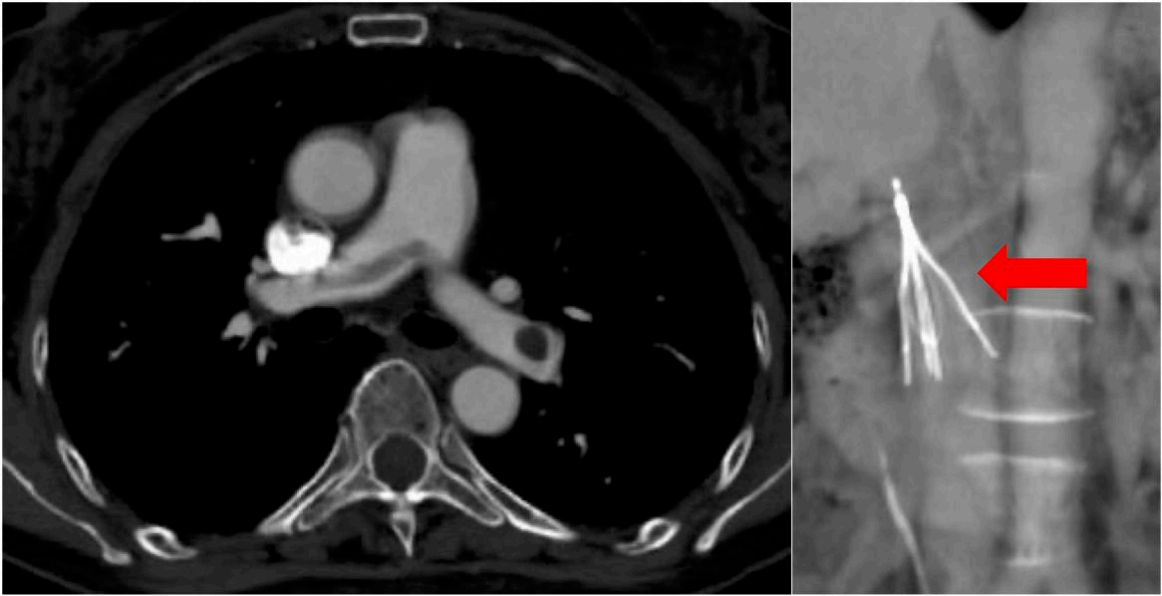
Contrast-enhanced computed tomography reveals a large thrombus in the pulmonary artery, and the previously observed thrombus in the inferior vena cava filter has disappeared. Additionally, the inferior vena cava filter is tilted.
